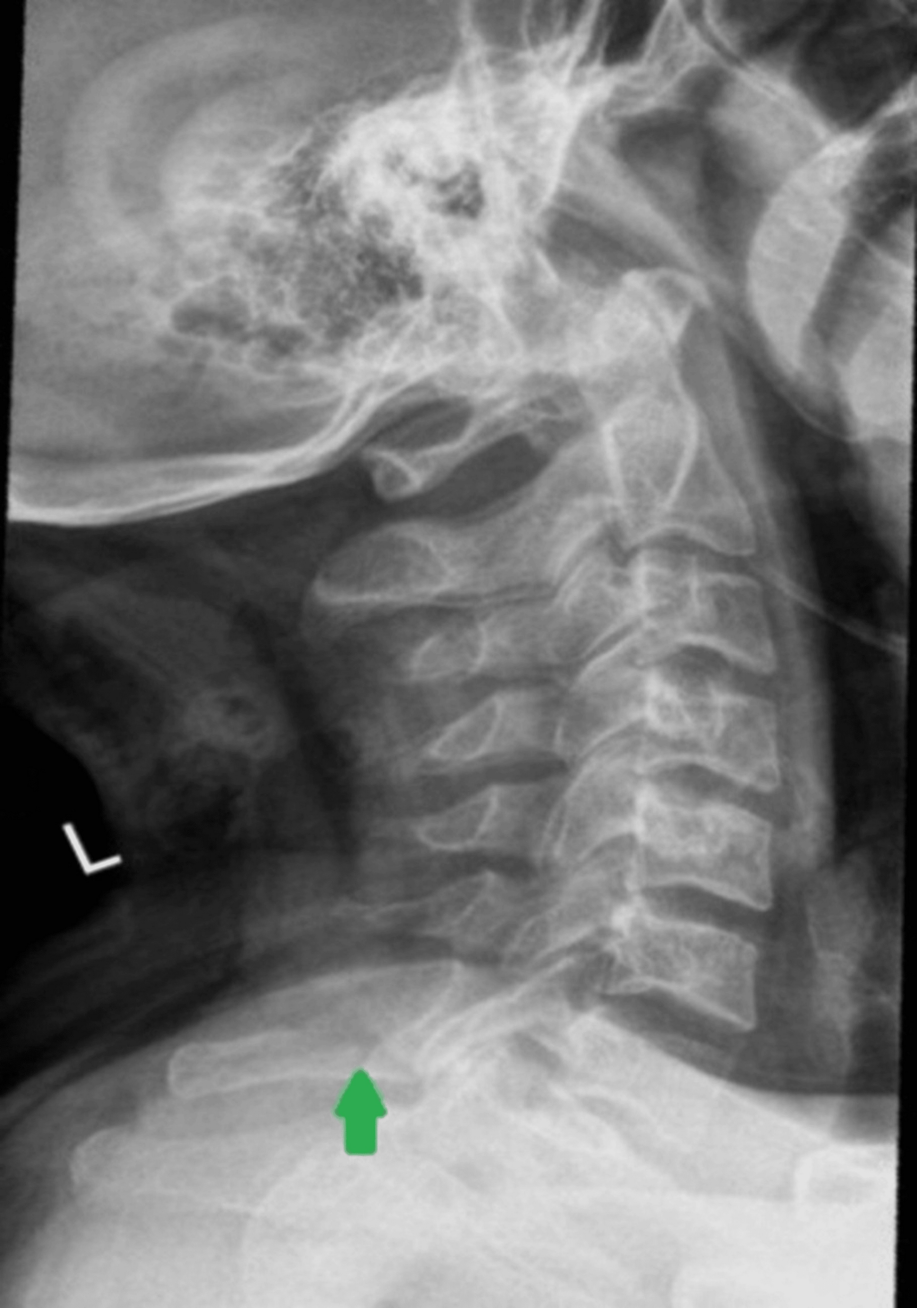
OPD follow-up cervical spine X-ray (lateral view) demonstrating the previously diagnosed C7 spinous process fracture without any interval change in the alignment or appearance of the fracture. OPD: outpatient department
